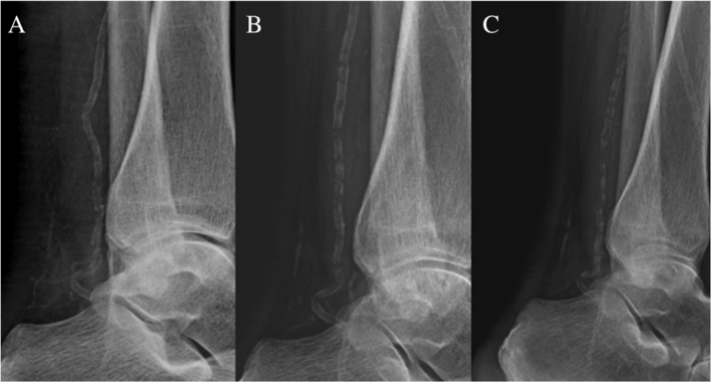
Plain radiographs of the left lower leg and ankle. (A) Six weeks after the onset of skin lesions, substantial calcifications were visible in the posterior tibial artery and a network of small vessels in the retromalleolar region, near the skin lesions. (B) After 11 months, the vessel calcification in the retromalleolar region was almost completely resolved and calcification in the posterior tibial artery showed apparent regression. (C) After a subsequent 11 months of cinacalcet treatment, the posterior tibial artery demonstrated further calcification regression.
